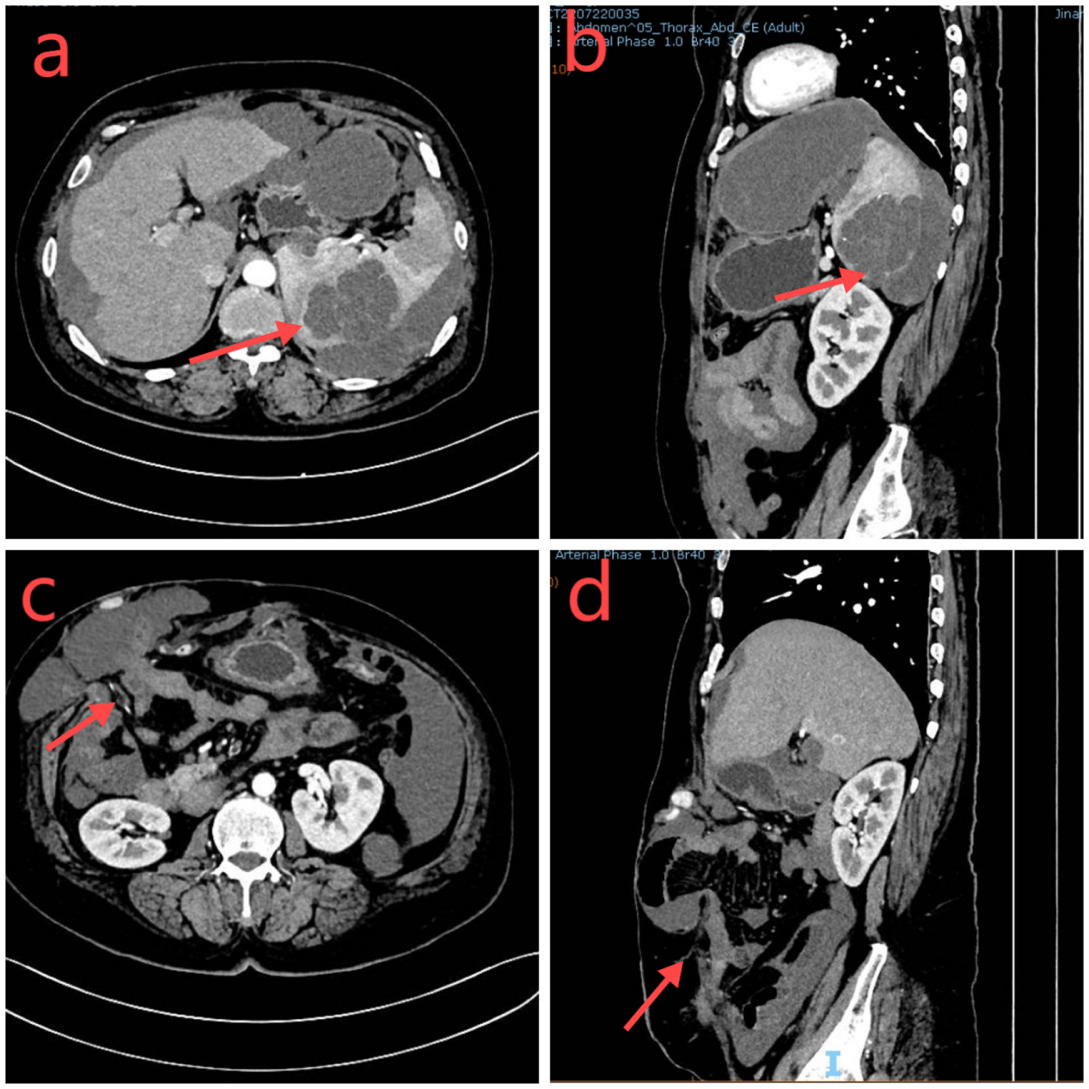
(a,b) The abdominal CT axial and sagittal sections demonstrate a low-density occupying lesion in the spleen (red arrow). (c,d): Adjacent to the original colostomy site, there is a herniation of the small intestine and surrounding tissues (highlighted in red).
